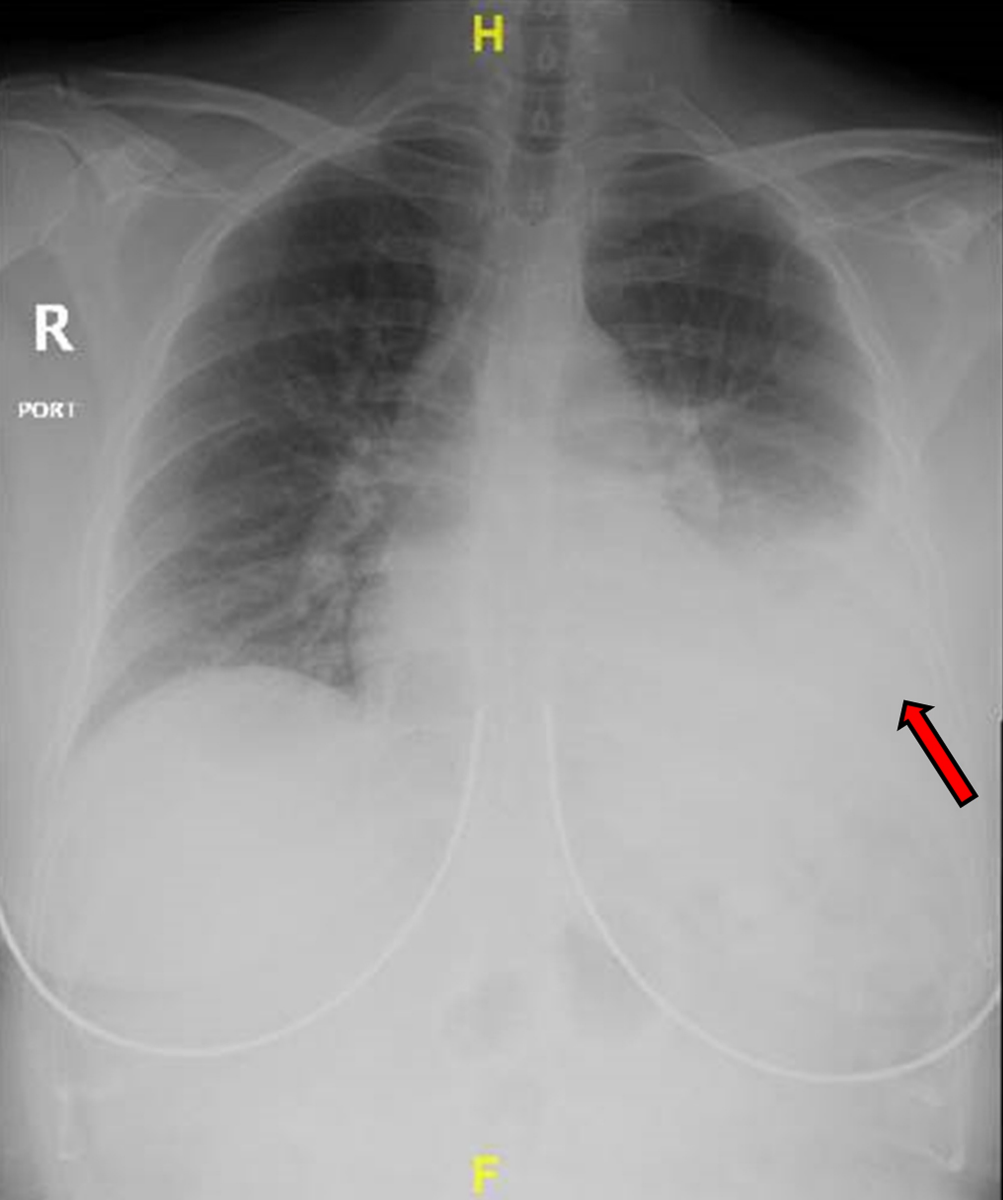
Chest X-ray showing moderate left pleural effusion (red arrow).
